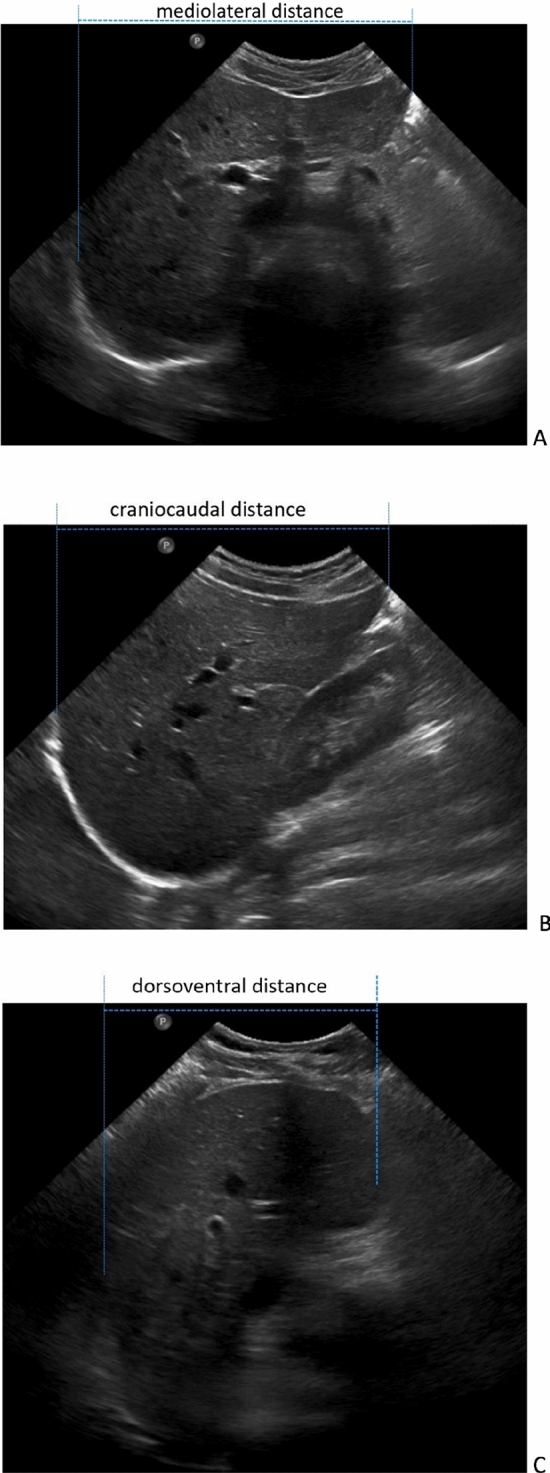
Example of a representative measurement of diameters in ultrasonography. Images were taken during the measurement of maximum diameters in (A) mediolateral (USmaxML), (B) craniocaudal (USmaxCC) and (C) dorsoventral direction (USmaxDV).
